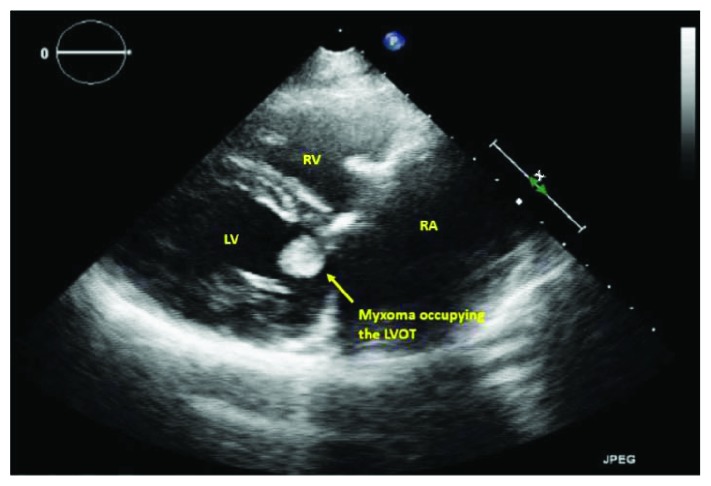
Transthoracic echocardiogram modified parasternal long-axis view showing a myxoma in the left ventricular outflow tract.
